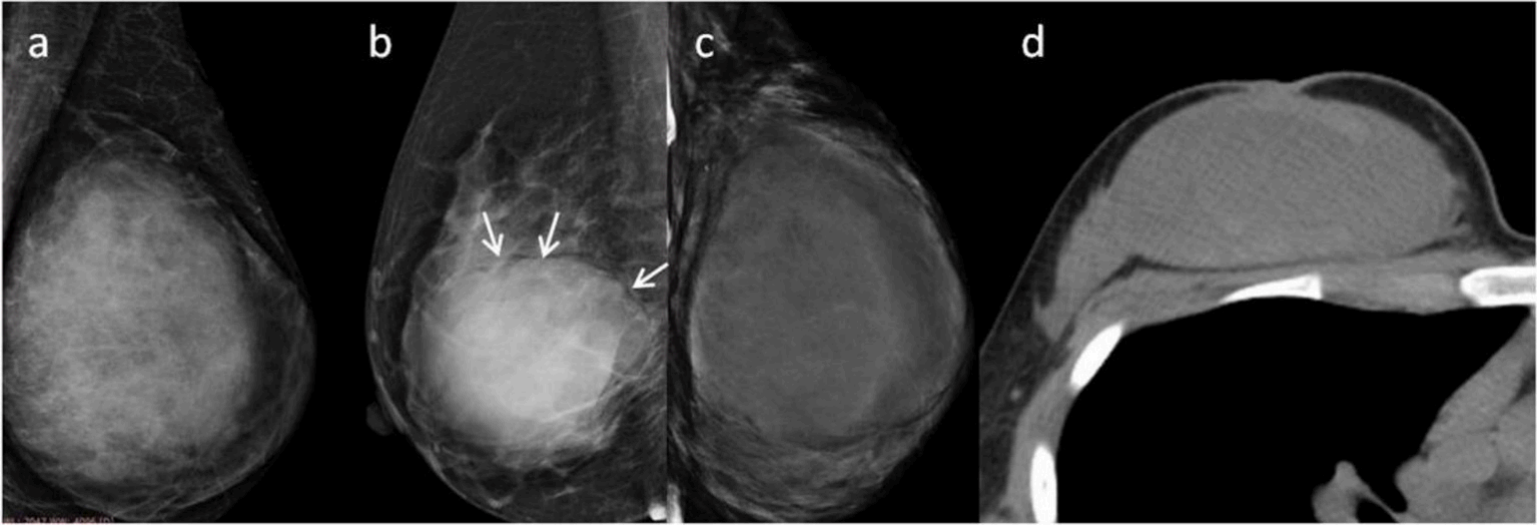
(a) Malignant Phyllodes tumor (PT) of left breast in a 55-year-old woman. A mediolateral oblique mammogram shows a well-defined isodensity mass with a diameter of 13 cm. (b) Malignant PT of right breast in a 47-year-old woman. Mammogram shows a well-defined high-density mass with a diameter of 9 cm. The mass is partially surrounded by a lucent halo (arrows). (c,d) Malignant PT of left breast in a 38-year-old woman. CT can show cystic changes within the tumor. They are all well-defined masses with large size.
